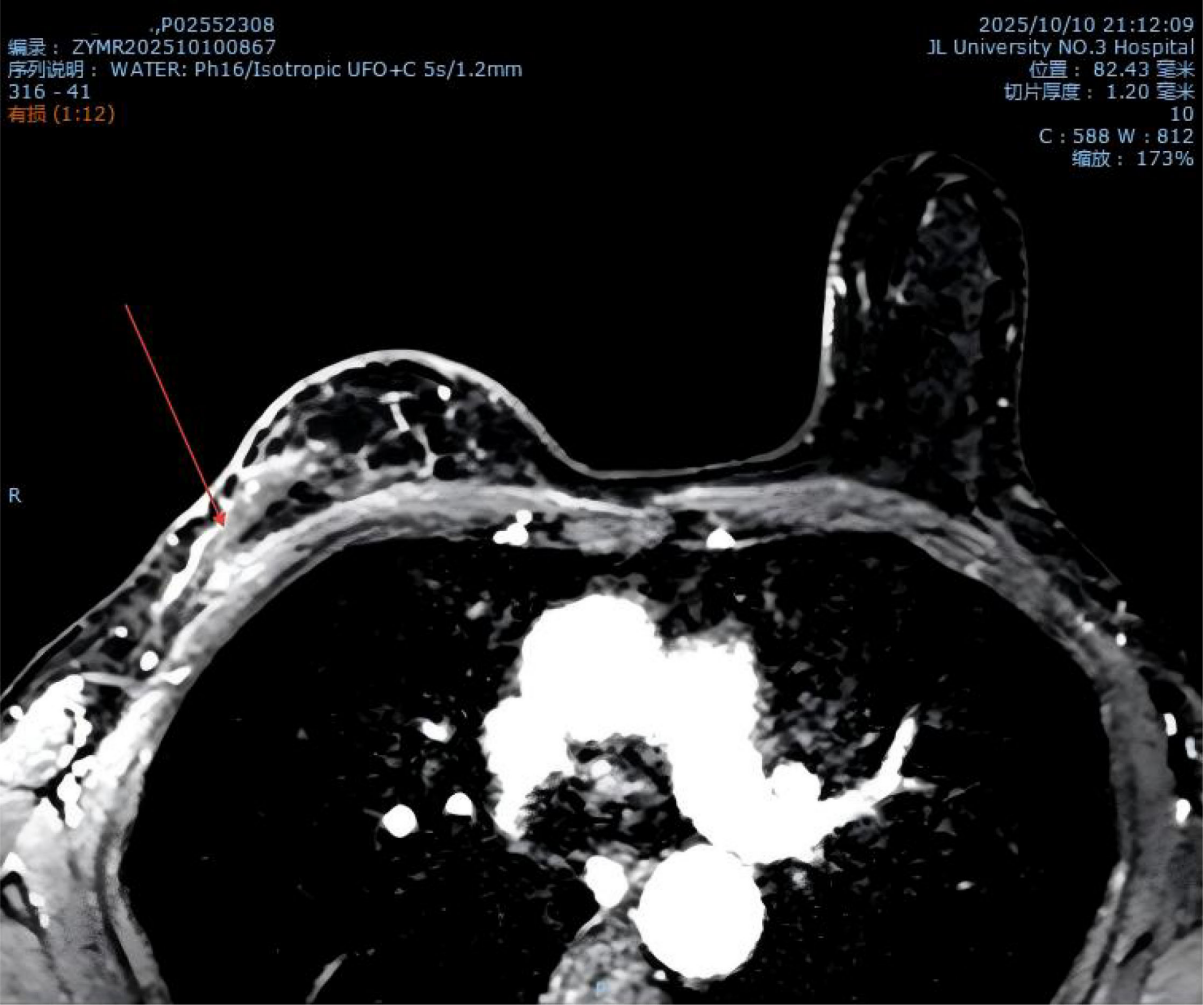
MR image from October 2025.
